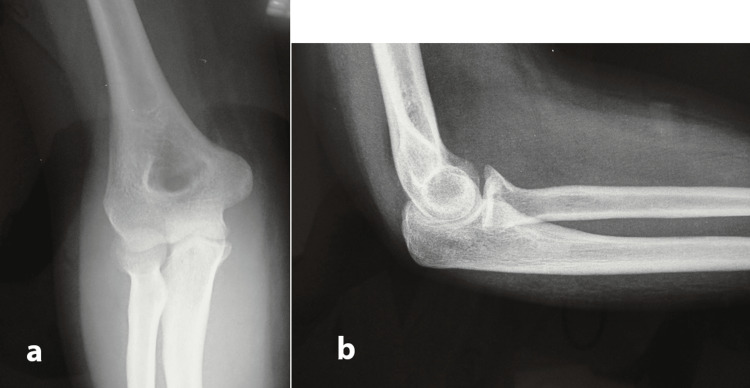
Three months post-injury anteroposterior (a) and lateral elbow (b) x-rays. Complete fracture healing noted on the coronoid process fracture.
